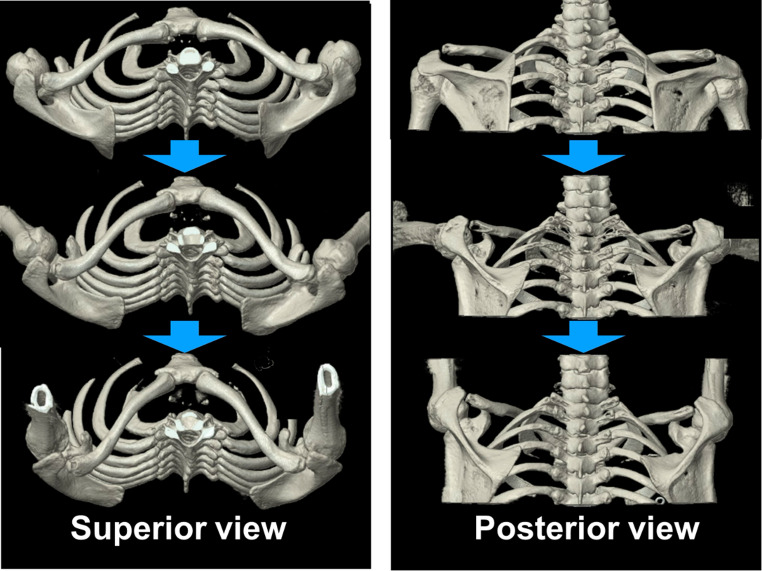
Upright four-dimensional CT images of shoulder motion. Superior (axial) and posterior (coronal) views demonstrate dynamic shoulder motion during arm elevation, enabling in vivo analysis of shoulder kinematics under physiologic load
